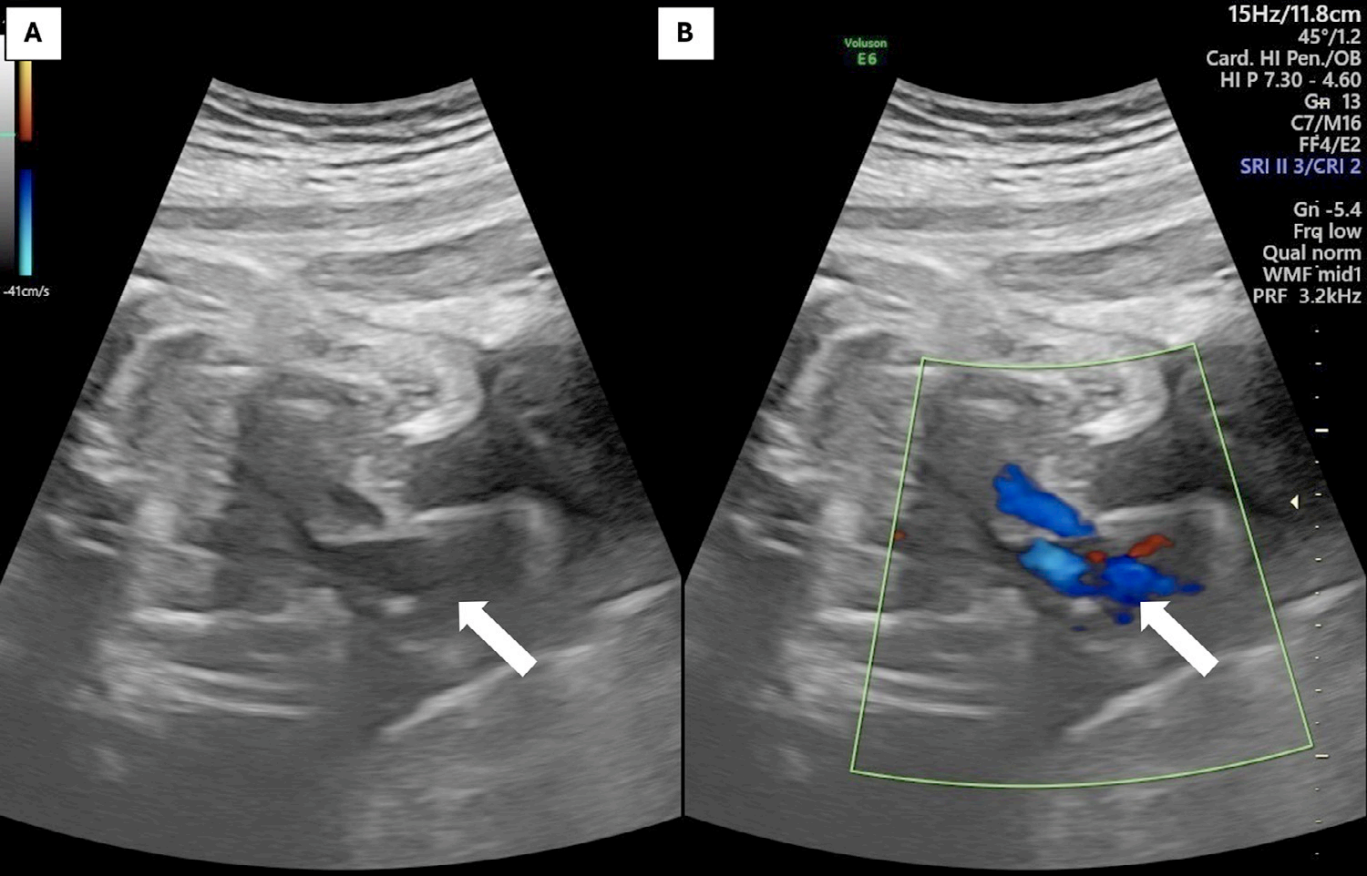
An ultrasound anomaly scan of a 36-week fetus with ectopic cordis reveals the heart entirely located outside the chest cavity (white arrow).
